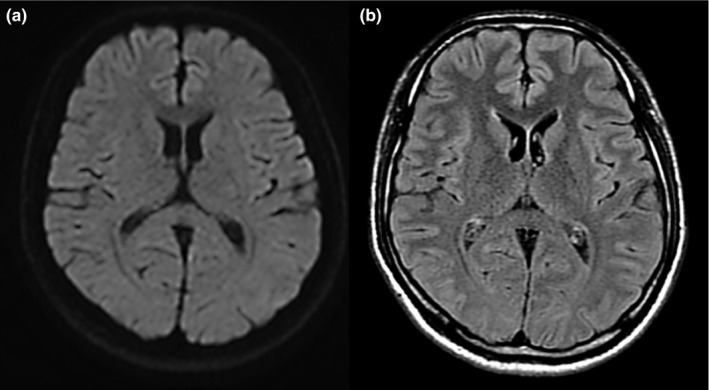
Magnetic resonance images on day 10 of hospitalization of the patient described in Figure 2. Axial diffusion‐weighted image (a) and fluid‐attenuated inversion recovery image (b) showed either disappearance or remarkable shrinking of the previously observed abnormalities. The patient was discharged without sequelae or medication
